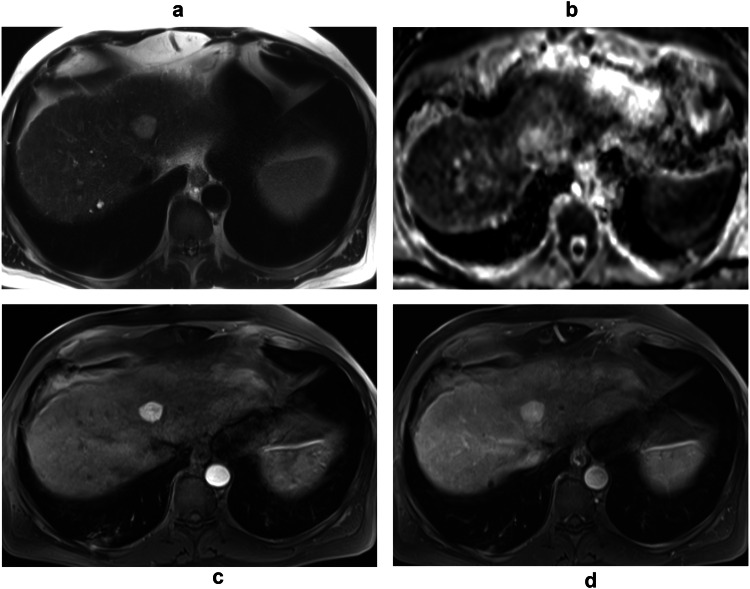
MRI of a 56-year-old male shows an atypical LGVNL pattern. Axial (a) T2-weighted image, (b) ADC map and (c, d) contrast-enhanced MRI in the (c) hepatic arterial and (d) portal venous phases show a lesion of the left lobe with moderate T2 hyper-intensity, no restriction on ADC and homogenous arterial phase enhancement that persists in the portal phase
